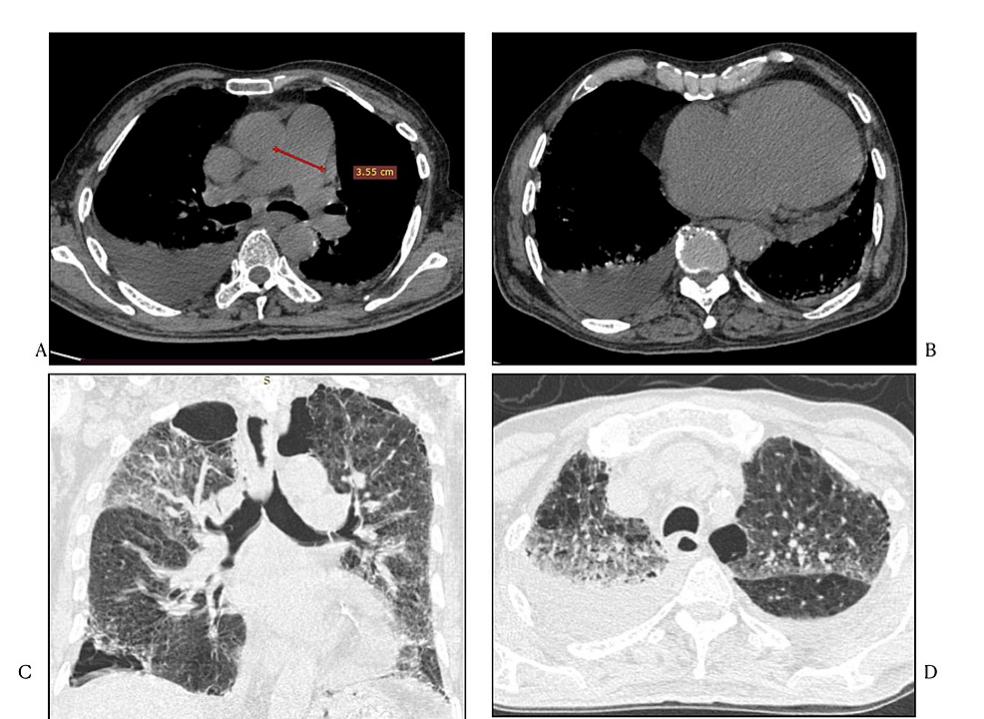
HRCT Chest (mediastinal and lung windows) in axial and coronal reformatted images A) Dilated main pulmonary artery (red line) and moderate right pleural effusion B) Cardiomegaly with minimal pericardial effusion C) & D) Peripheral ground glass opacities with background emphysematous changes
